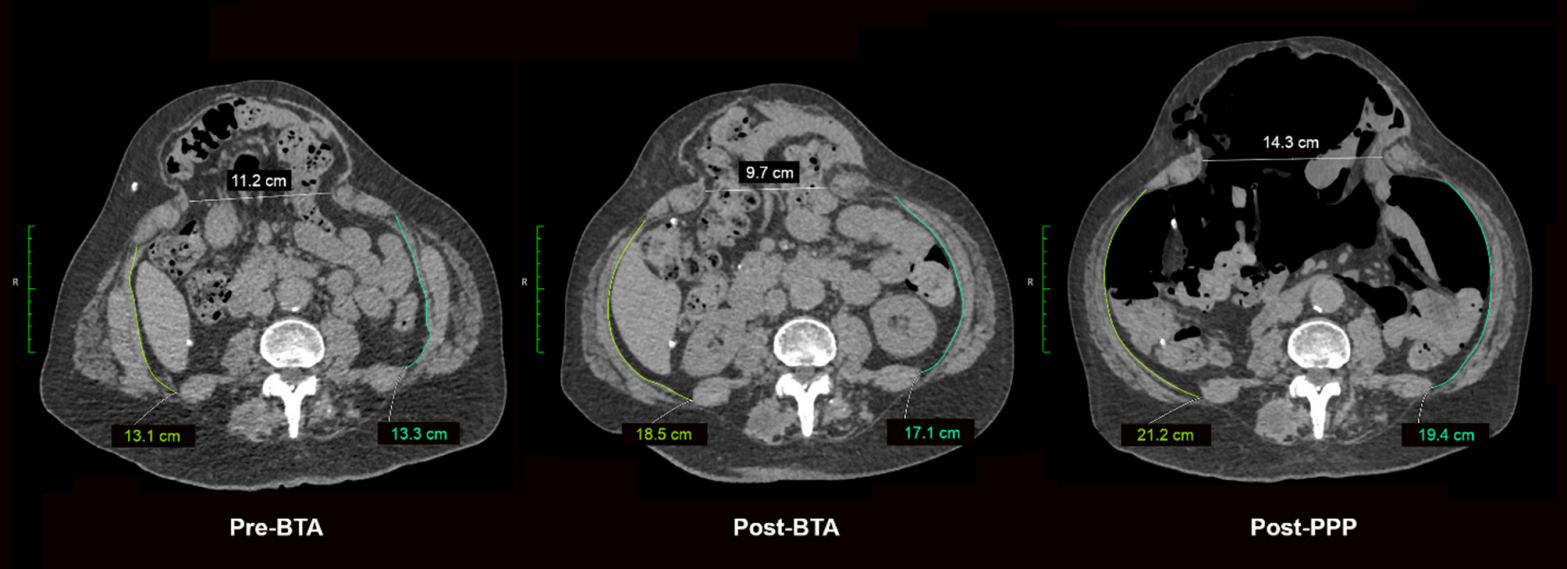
Axial CT images demonstrating progressive changes in abdominal circumference and hernia defect measurement changes (Baseline/Pre-BTA, Post-BTA and Post PPP). Measurements taken from the same vertebral level on each occasion.
